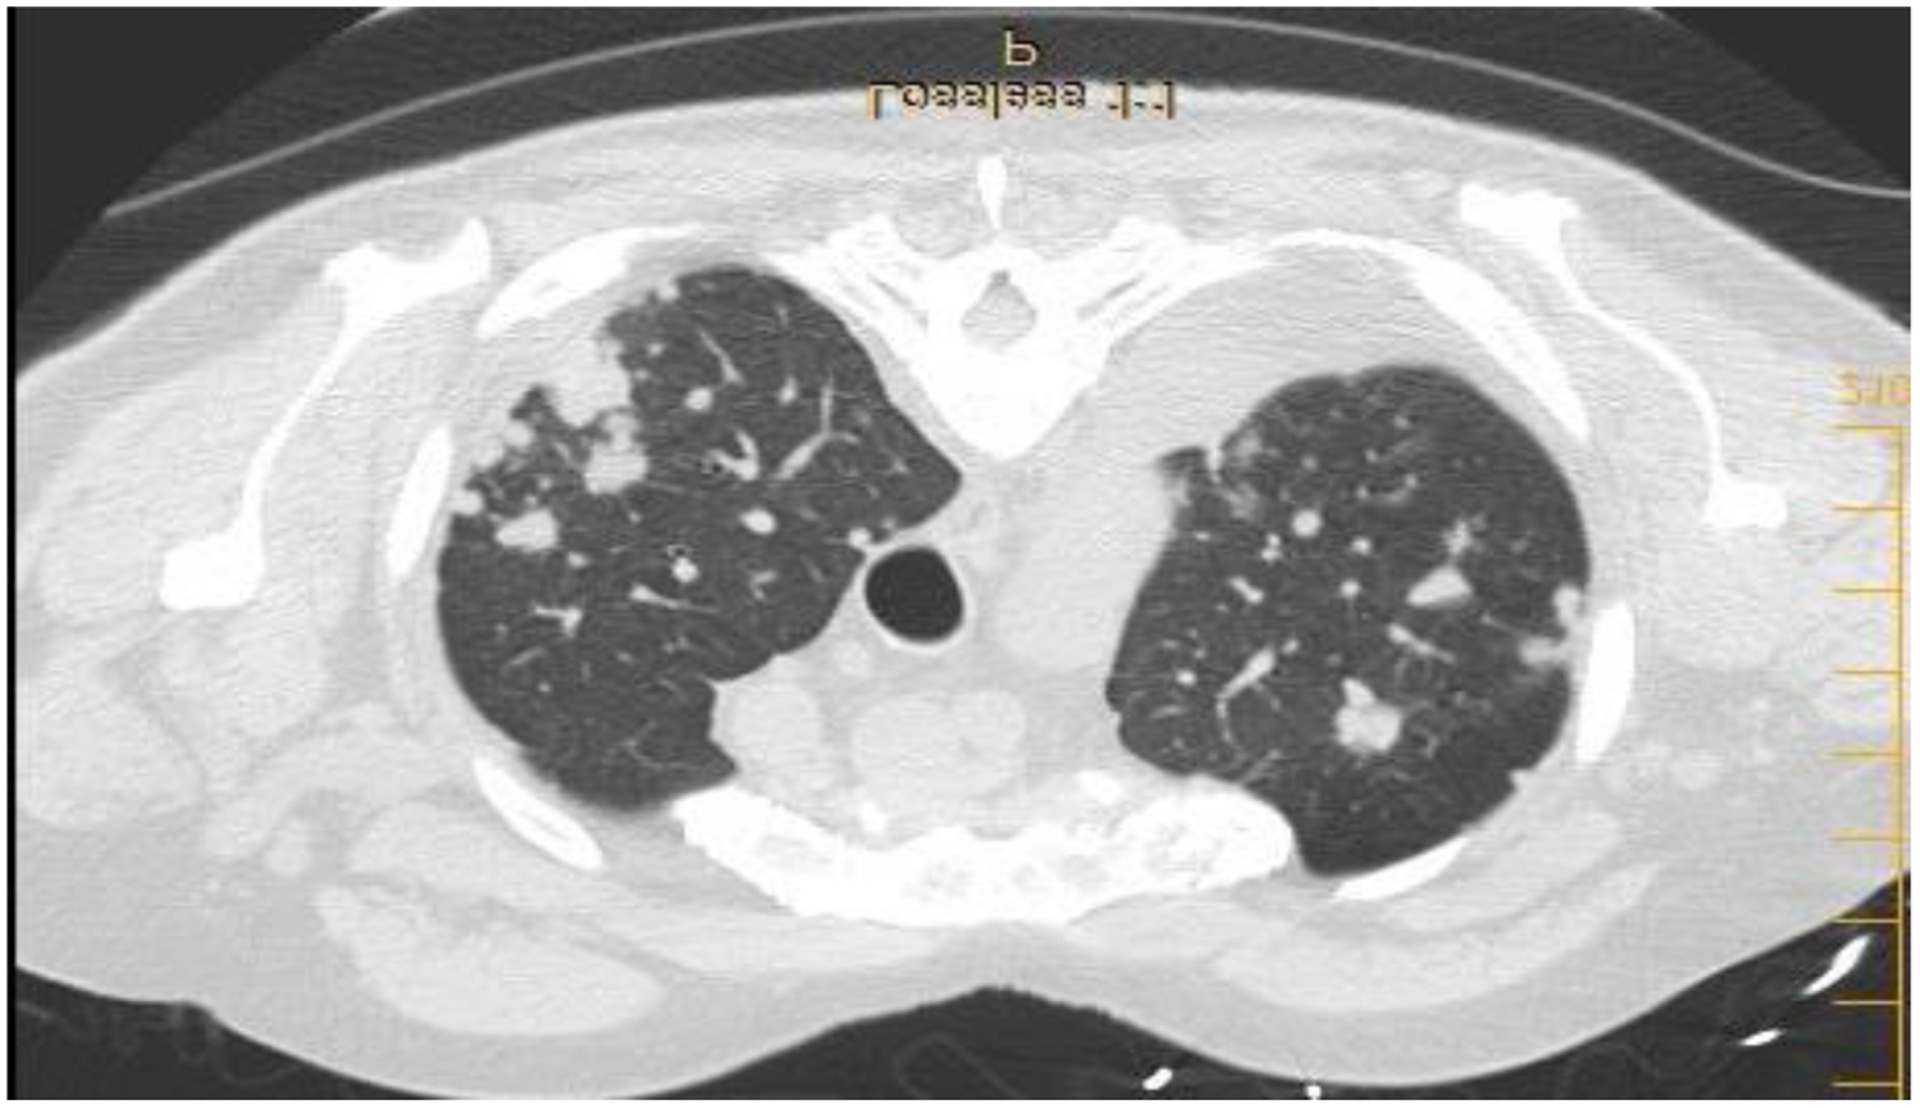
Axial CT chest showing multiple pulmonary nodules in the right upper lobe. Black arrow pointing to RUL nodules
